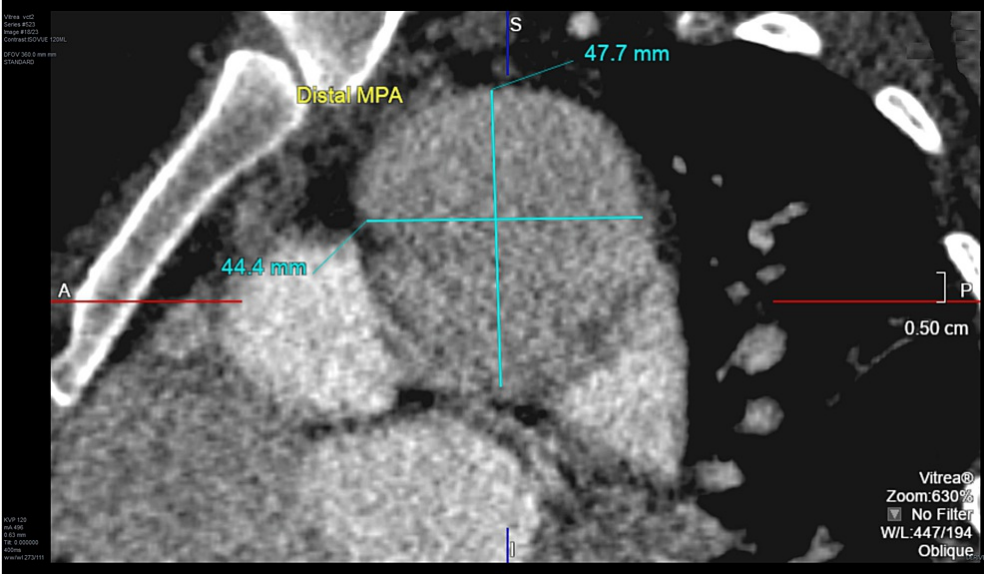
Aneurysm of the main pulmonary artery on computed tomography angiogram of the chest.
